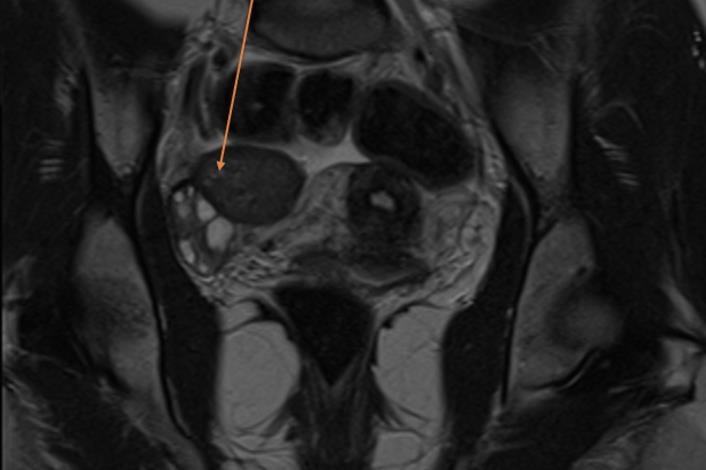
MRI appearance of ovary.
